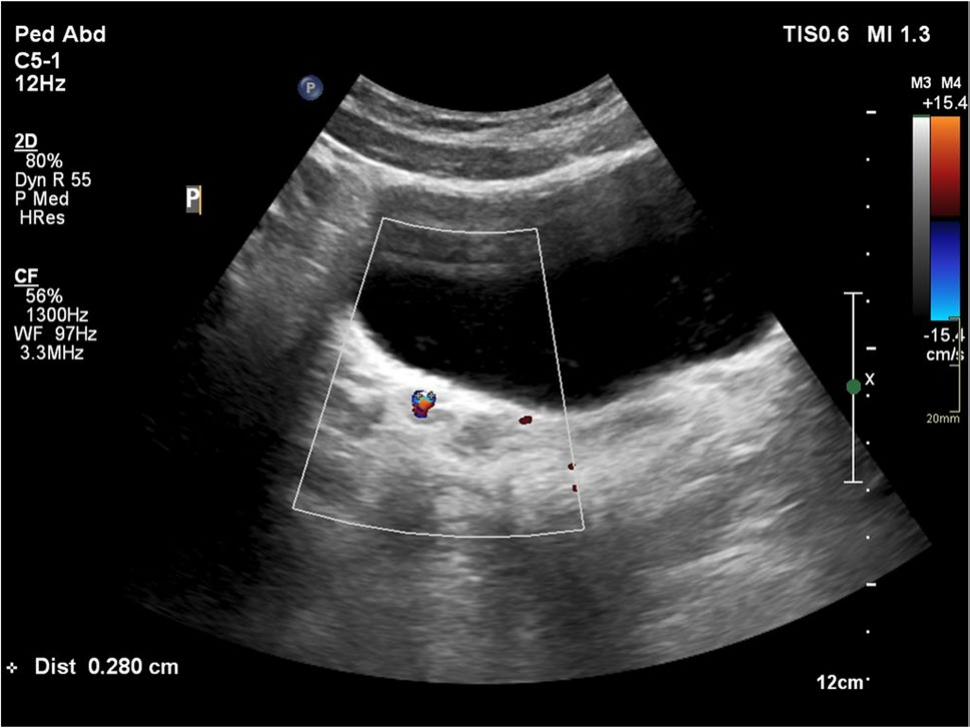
Colour Doppler axial image of the bladder of a 17-year-old girl who presented with right lower quadrant pain and haematuria shows a stone in the distal part of the right ureter. Twinkling artifact facilitates differentiation of the stone from other echogenic structures
